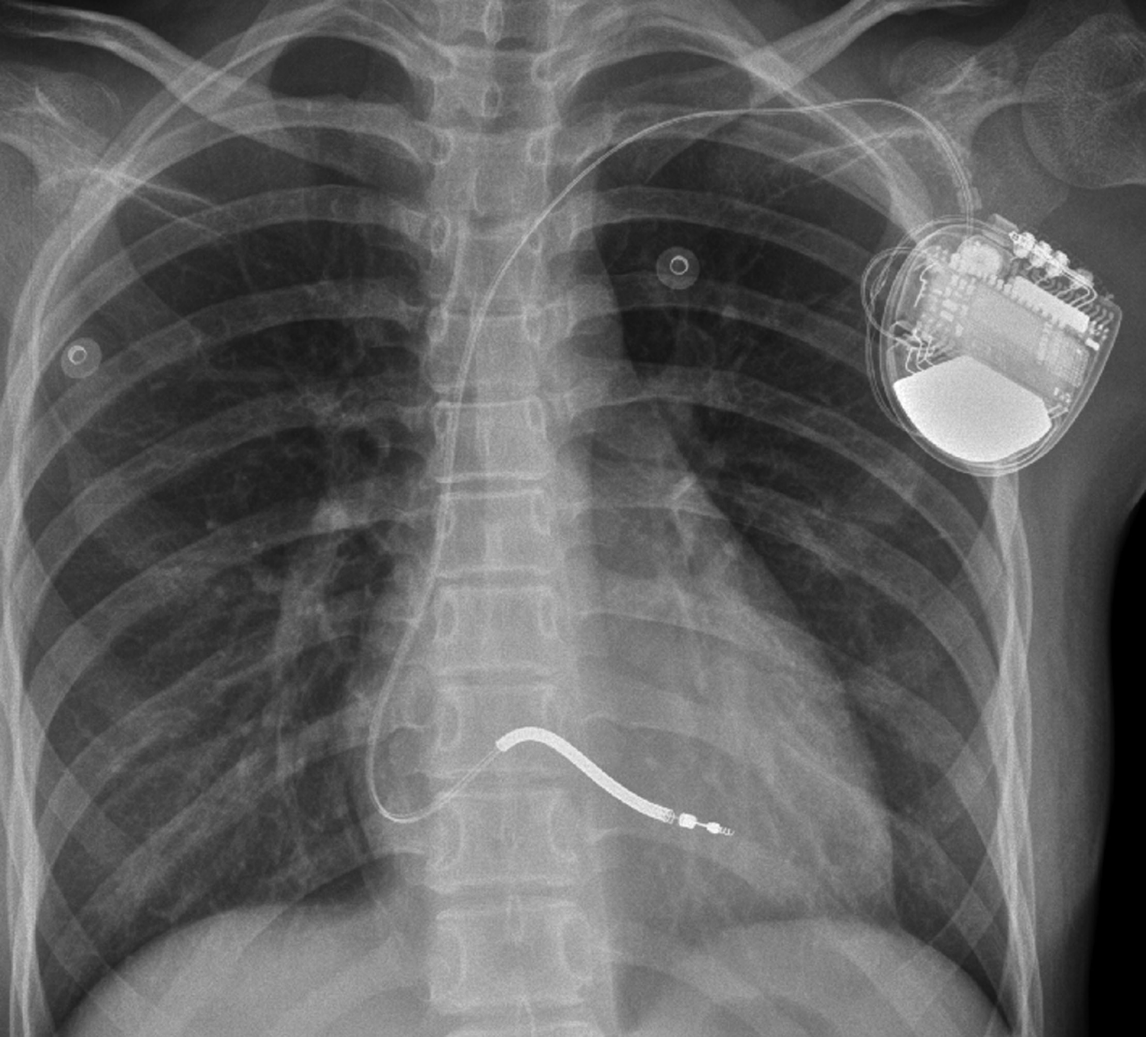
Chest x-ray after the implantation of the implantable cardioverter-defibrillator.
